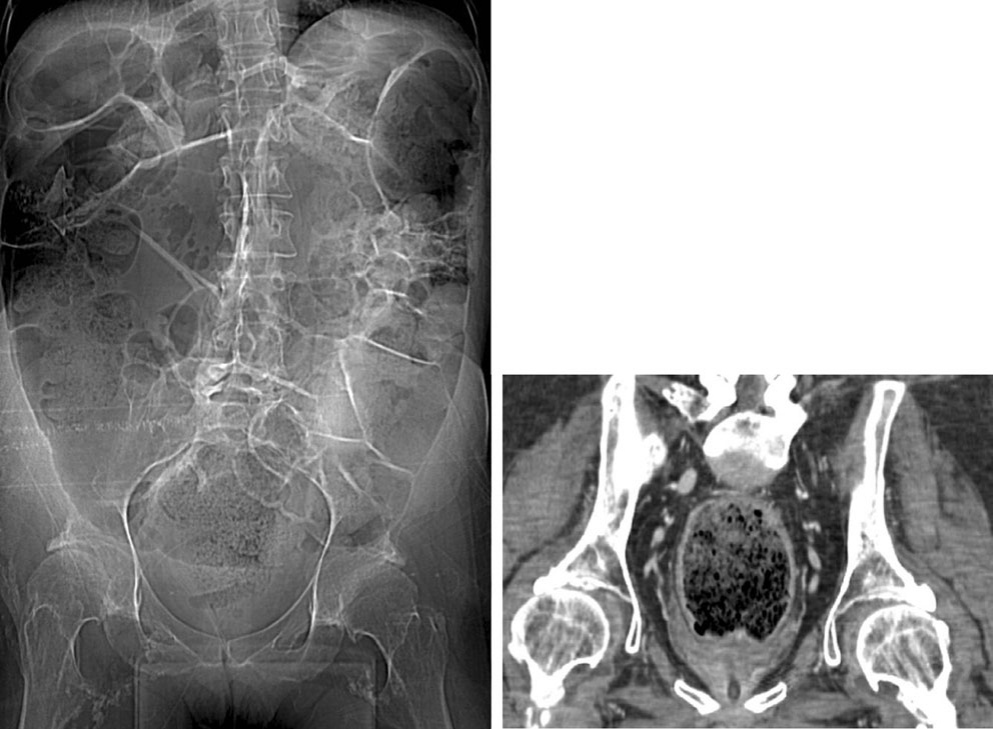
Faecal impaction: scout radiograph of the abdomen demonstrates a large volume of stool throughout the colon and rectum. Coronal CT image confirms large rectal stool ball with mild associated rectal wall thickening, compatible with faecal impaction
